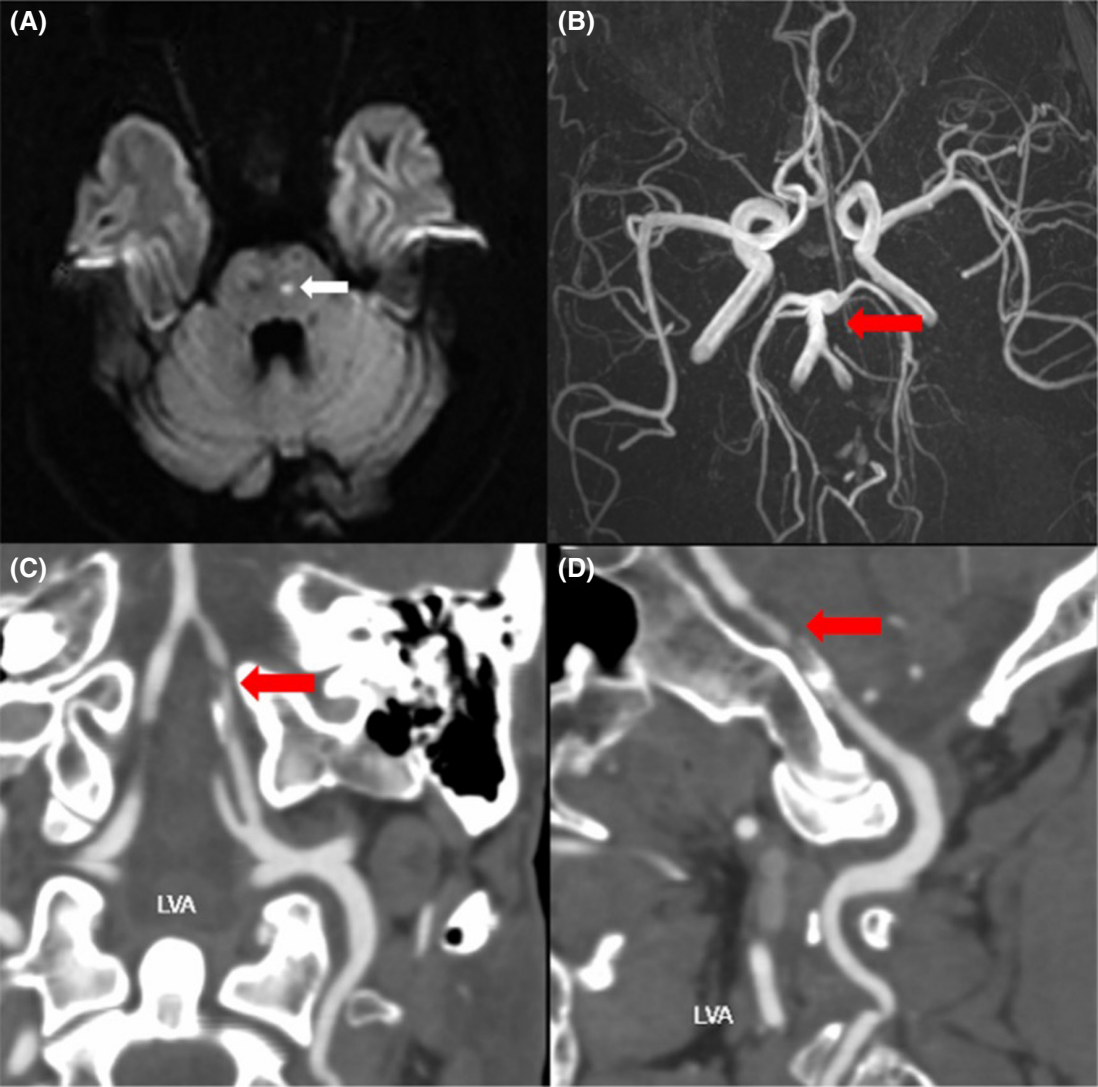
Brain imaging presentations. (A) MRI DWI revealed an acute lacunar infarct on the left side of the pontine brain and lacunar foci on the right side of the pontine brain (white arrow). (B) Brain MRA and (C‐D) CTA showed bilateral vertebral artery stenosis, with the left side predominant (the high likelihood of a dissecting aneurysm; red arrow). Abbreviation: CTA, computed tomography angiography; DWI, diffusion‐weighted imaging; MRA, MR angiography; MRI, magnetic resonance imaging
